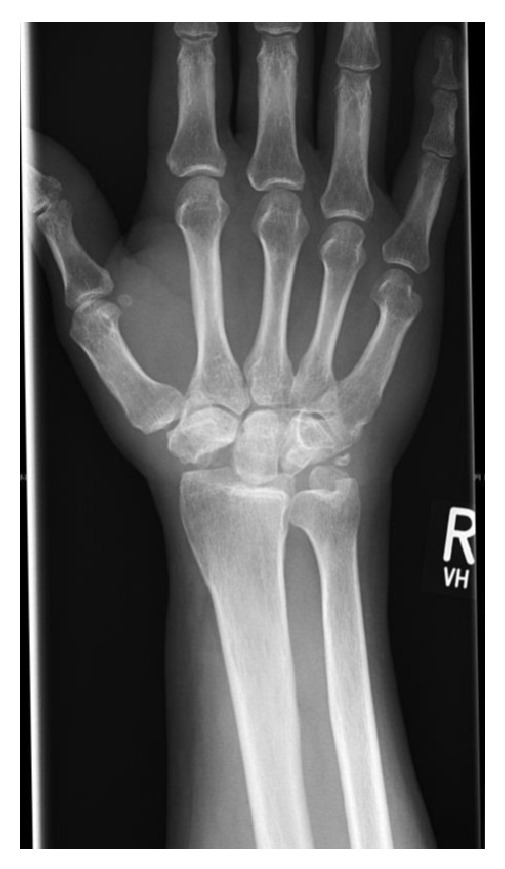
X-ray: postproximal row carpectomy.
